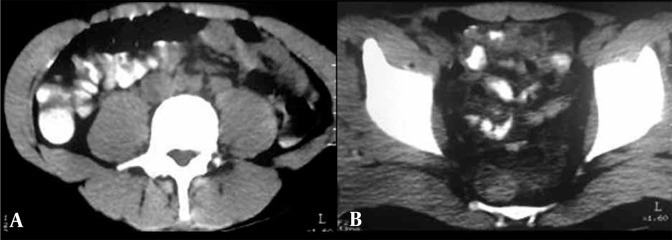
A-B, Non-contrast CT shows hyperdense material mixed with bowel contents with no history of prior barium or other contrast study. The findings are consistent with opened opium packets.
